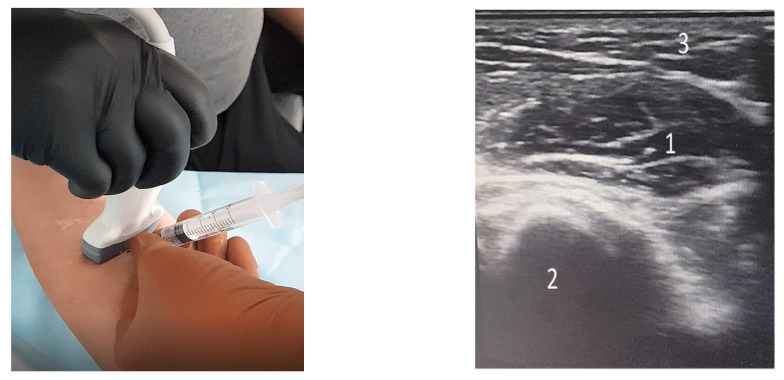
Incobotulinumtoxin A injection of the right upper limb—pronator teres muscle. The ultrasound image shows the pronator teres muscle (1), to be injected, and adjacent structure: ulna bone (2) and subcutaneous tissue (3). On the gray scale, the muscle appears more hypo echoic, bordered by hyper echoic lines, representing the muscular fascia. Ulna bone—only the edge that appears intense hyper echoic-white is visualized, the rest of the bone structure not being visible by ultrasound and the shadow cone appears-black-under the bone. The subcutaneous tissue is hypo echoic and located on the surface, above the muscle.
